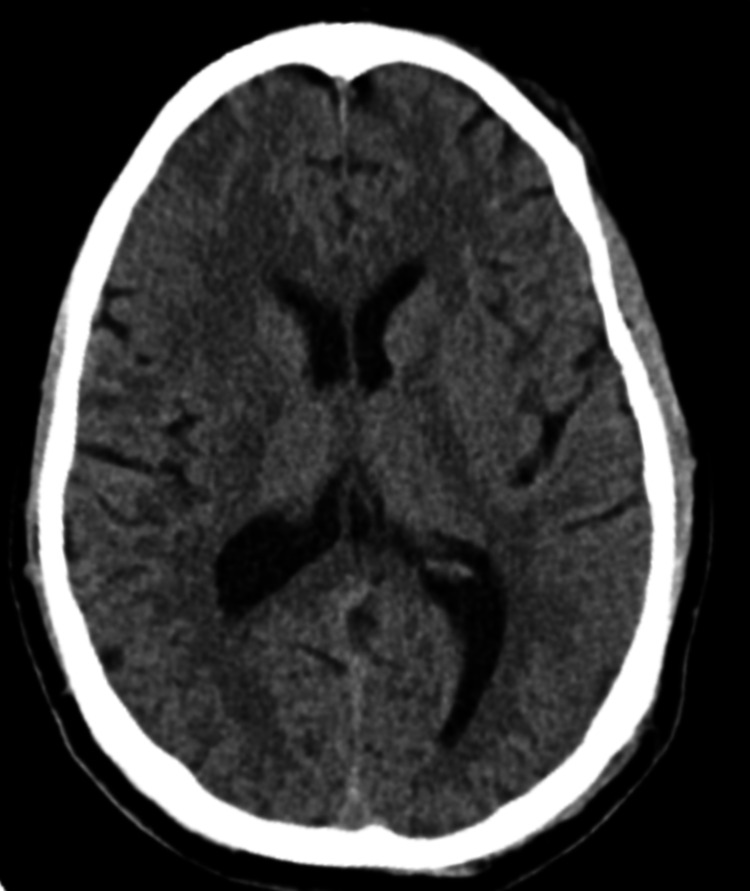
Axial view of the non-contrast CT scan of the brain The image showed no acute intracranial findings CT: computed tomography
